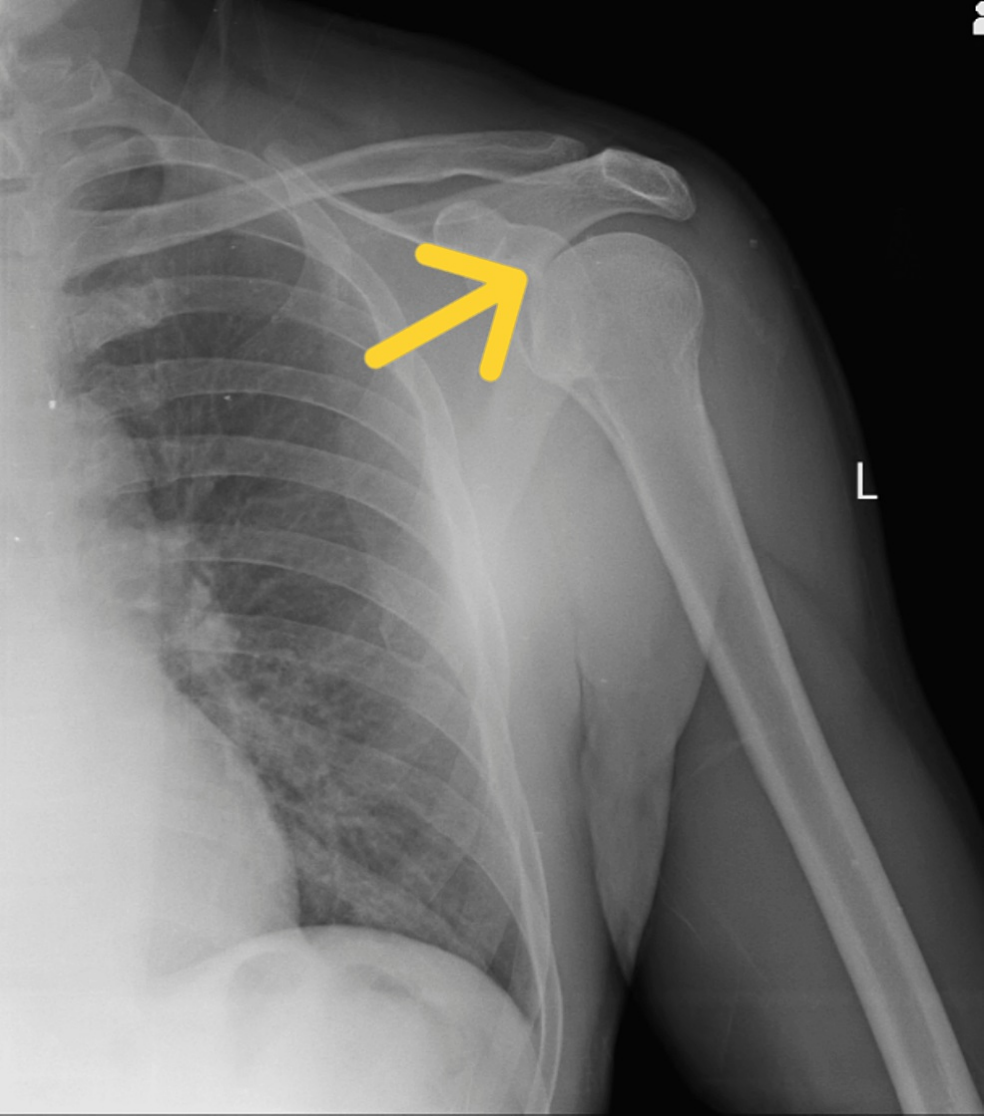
X-ray showing the anterior humerus dislocation The yellow arrow shows the site of dislocation.
